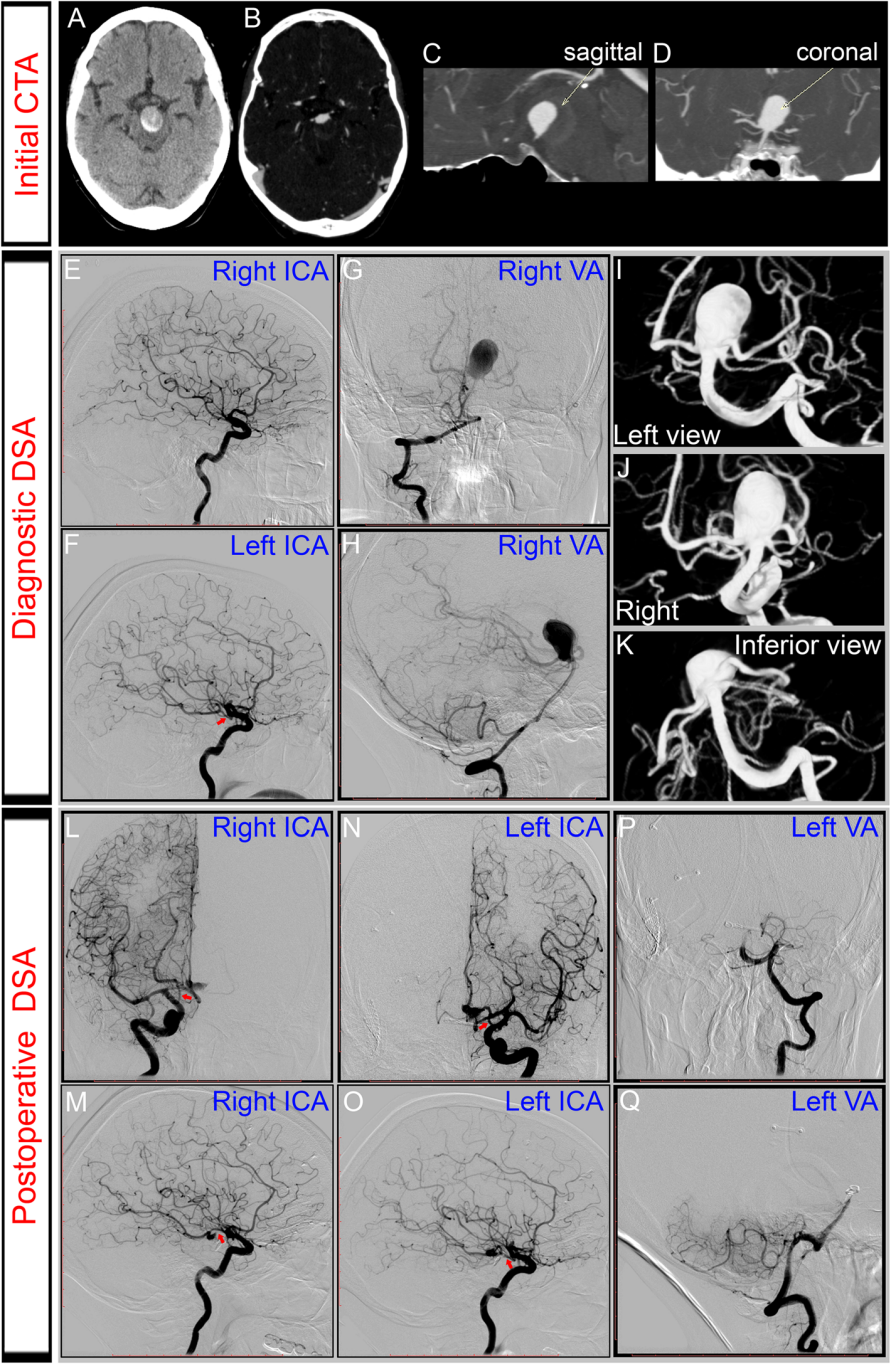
Preoperative computed tomography (CT) and digital subtraction angiography (DSA) demonstrated a basilar apex giant aneurysm occupying the interpeduncular fossa. a-d: Plain and contrast enhanced CT showed a giant calcified basilar artery aneurysm causing brain-stem compression. e-k: Diagnostic DSA with a right vertebral artery (VA) injection showing a basilar artery (BA) tip aneurysm. The diameters of the blood lumen measured 2.5 × 1.5 cm. The aneurism projected posteriorly into the interpeduncular cistern. A left robust PCoA was observed (red arrow in f). Based on the angiographic anatomy, trial balloon test occlusion was not required. Anteroposterior (g) and lateral (h) DSA with right VA injection showed partial filling of the aneurysm sac due to thrombosis. The aneurysm was wide-necked, with incorporation of both posterior cerebral arteries (PCAs) and superior cerebellar arteries (SCAs) into the neck (3D reconstructions, I-K). l-q: Postoperative DSA confirmed occlusion of the BA and filling of the top of the BA via the posterior communicating arteries (PCoAs) bilaterally (red arrows in L-O). DSA also revealed continued filling of the PCAs and SCAs through the PCoAs. Stagnant flow was also visible in the aneurysm, indicating a high likelihood of aneurysm thrombosis. In fact, there was a marked decrease in the diameter of the contrast filling in aneurysm blood lumen
